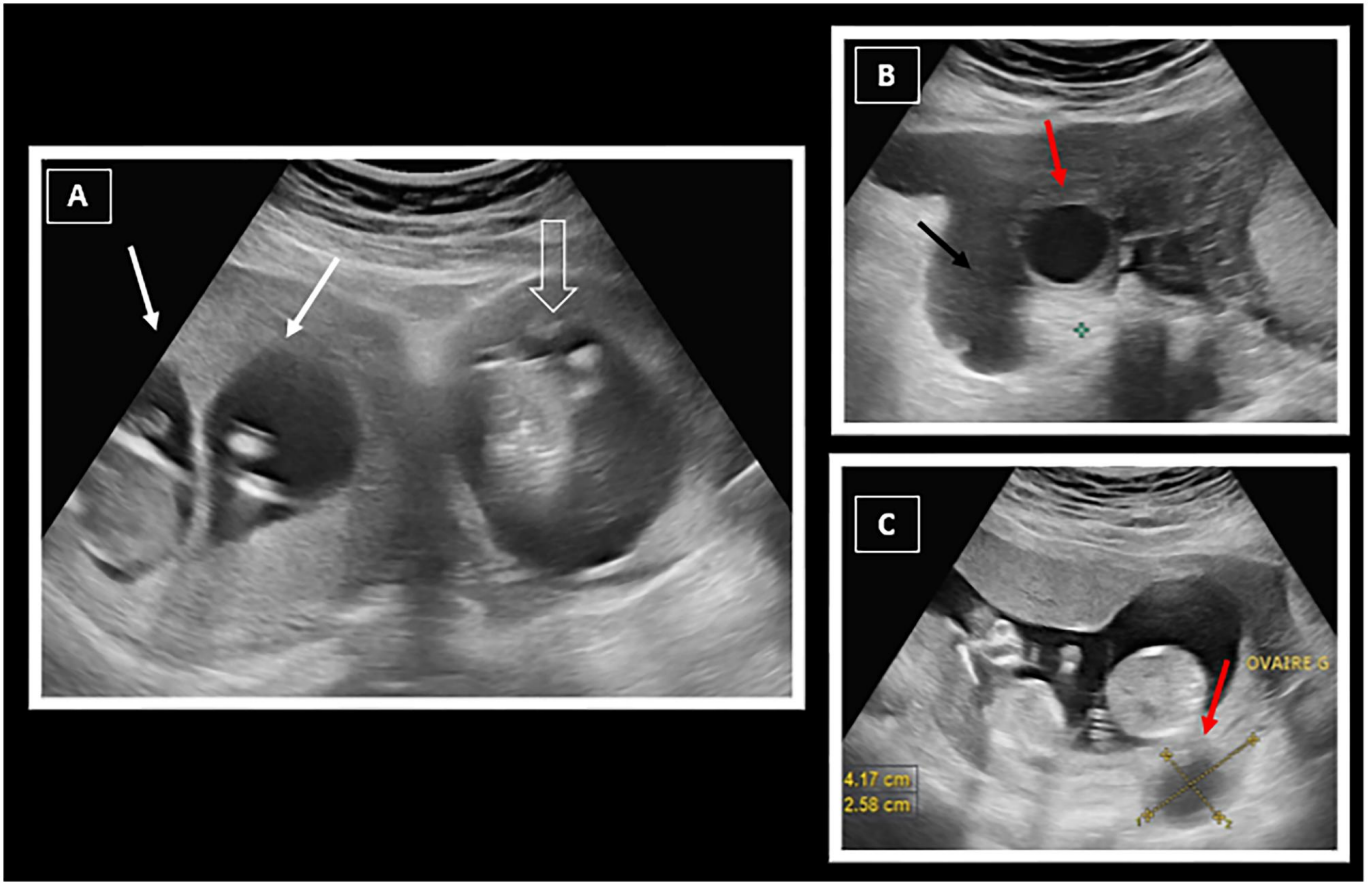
Trans-abdominal ultrasound images obtained using a low-frequency convex probe showing an evolving triplet pregnancy. (A) Two gestational sacs are seen in the uterine cavity (white arrows), while a third adjacent gestational sac is lateralized to the left, but does not appear to be developing within the uterus (empty arrow). (B and C) Enlarged and follicular right (B) and left (C) ovaries are visualized on either side of the uterus, probably in relation to ovarian hyperstimulation syndrome (red arrows). Note the finely echogenic peritoneal effusion in (B), suggesting haemoperitoneum (black arrow).
